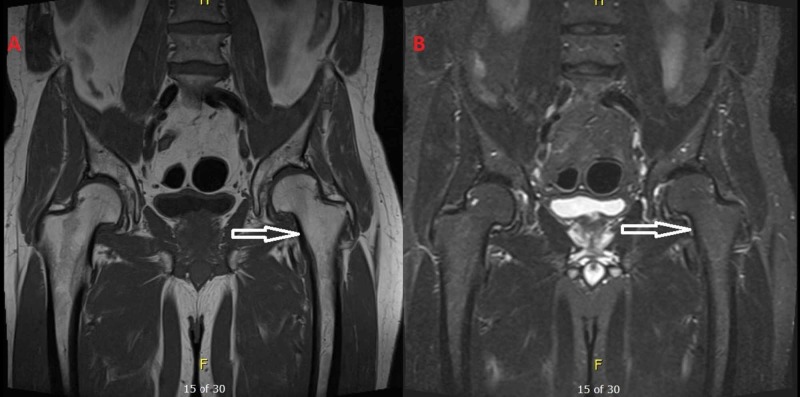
(A) T1-weighted images and (B) T2-weighted images from the MRI after the regression of the symptoms, five months after the initial diagnosis of bone marrow edema syndrome. The bone marrow edema has subsided.
